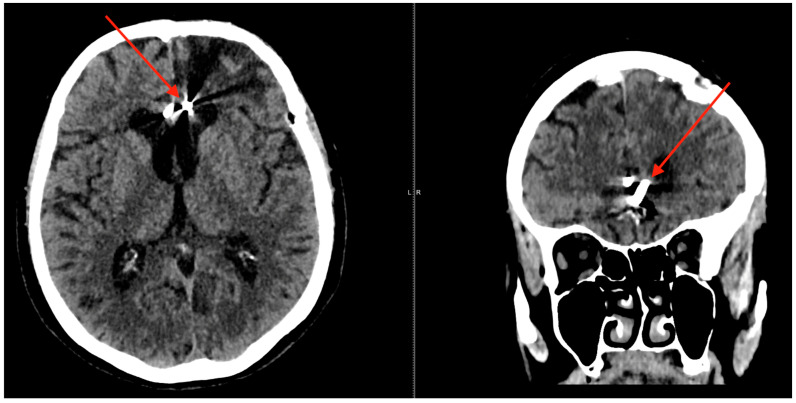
Follow-up control CT scan comparing transversal (left) and frontal (right) sections, showing the surgical clips within the anterior frontal interhemispheric and pericallosal cisterns. The red arrows mark the clip location in both views, confirming its precise and stable placement in the interhemispheric fissure, providing a clear spatial reference for post-operative evaluation.
